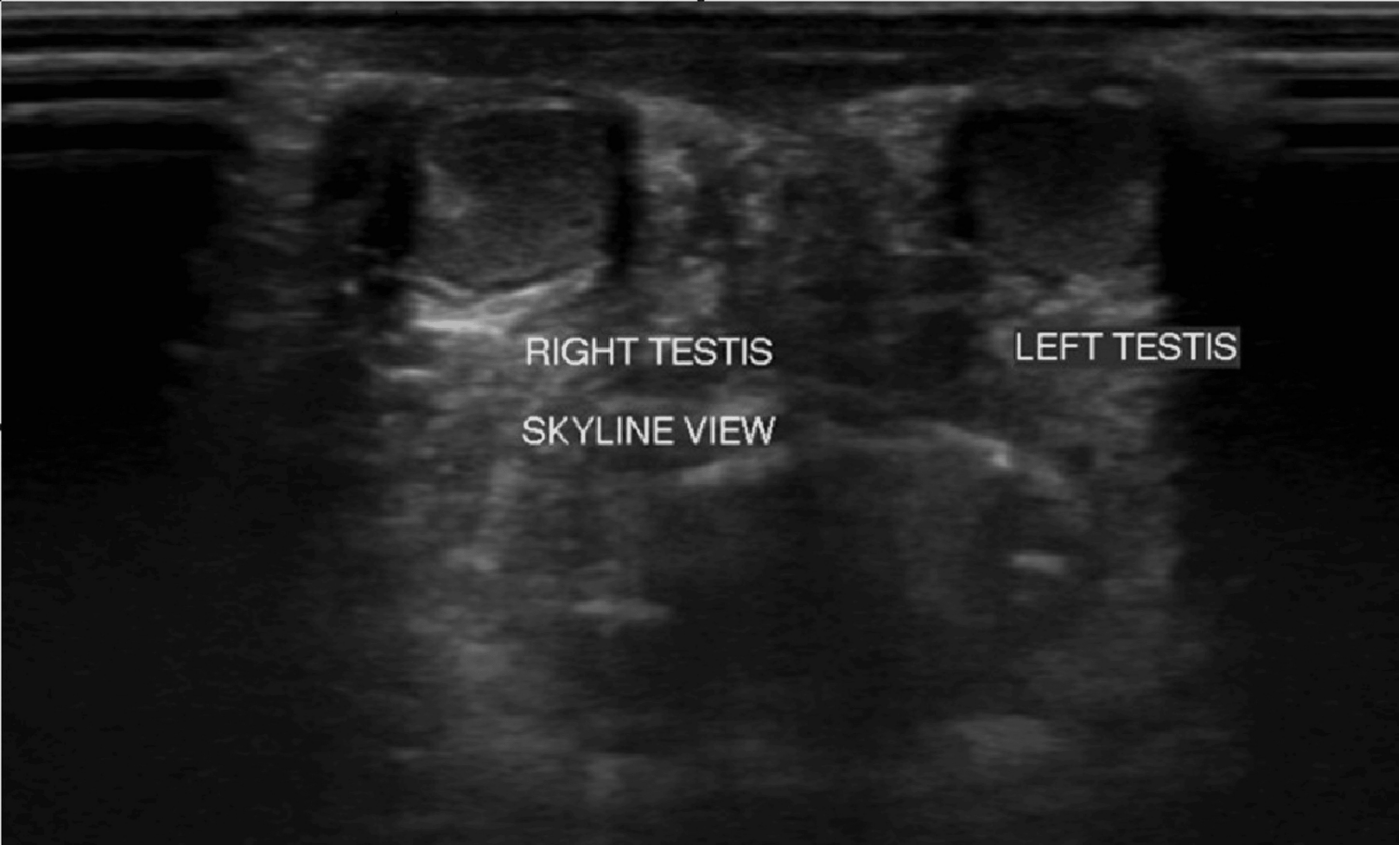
Ultrasound image showing skyline view showing both the atrophic testes.
